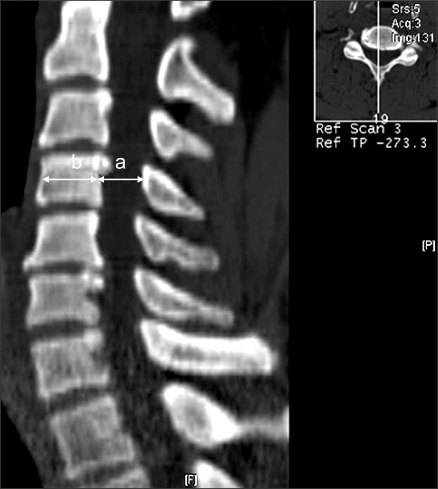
On the CT image, the diameters of the spinal canal (a) and vertebral body (b) are measured at the midvertebral level on sagittal-reconstructed CT images. The spinal canal/vertebral body ratio is determined using the formula a/b.
